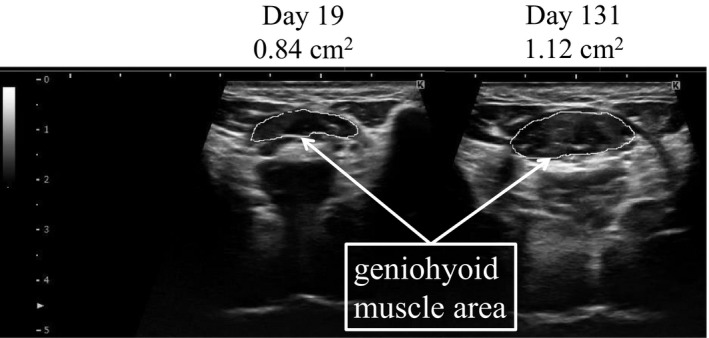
Coronal section of geniohyoid muscle area assessed by ultrasonography. Coronal section of geniohyoid muscle area increased by 33% from 0.84 cm^2^ at day 19 to 1.12 cm^2^ at day 131
